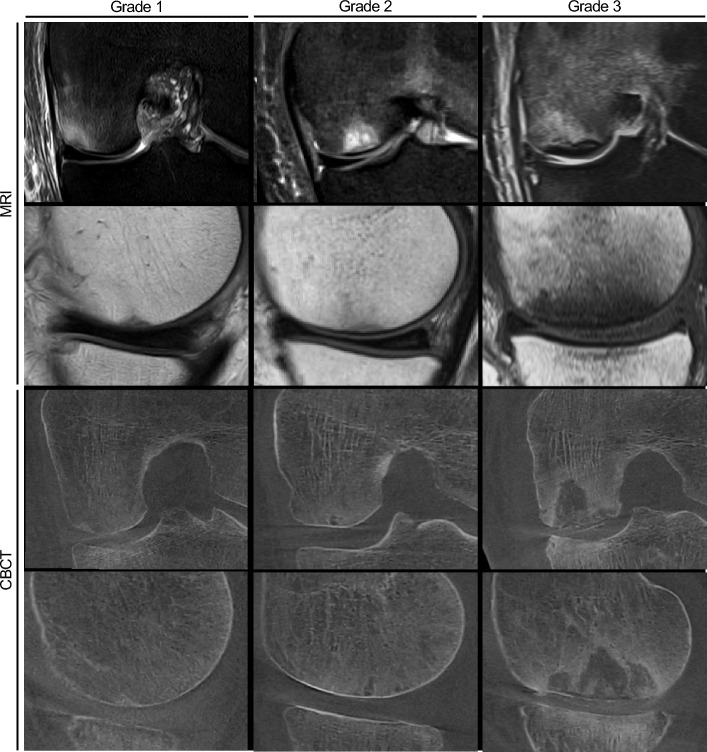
Grading of subchondral insufficiency fractures in MRI vs. CBCT. *MRI* magnetic resonance imaging; *CBCT* cone beam computed tomography. Grade 1: no visible subchondral bony lesion in MRI or CBCT. Bone marrow edema in the femoral condyle with elevated signal in PD (first row) and decreased signal in T1 MRI sequences (second row). Grade 2: subchondral fracture or cystic changes with circumscribed lesions of highly elevated signal in PD sequences without an infraction of the subchondral bone plate in CBCT. Grade 3: subchondral bone plate collapse and with corresponding cortical discontinuity of the cortical delimitation in T1 sequences and circumscribed overlying articular surface step offs in CBCT.
